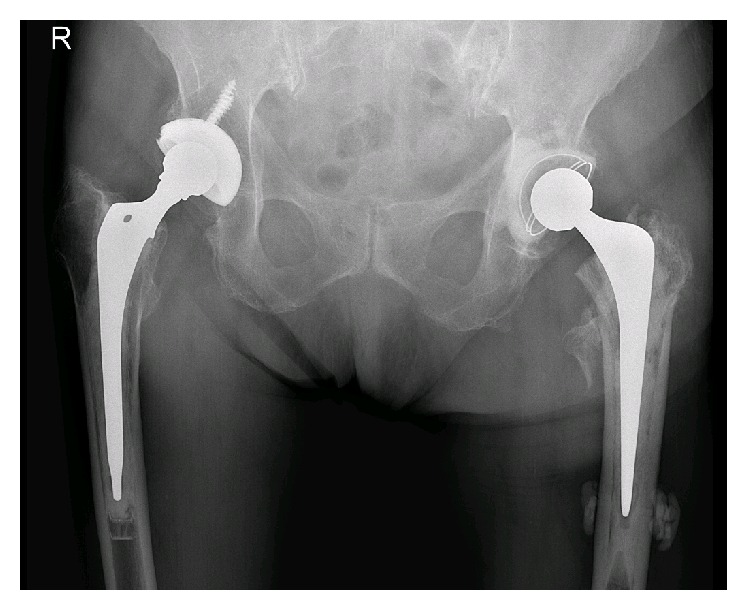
Anterior-posterior X-ray showing the cemented total hip arthroplasty after removal of the Gamma nail.
